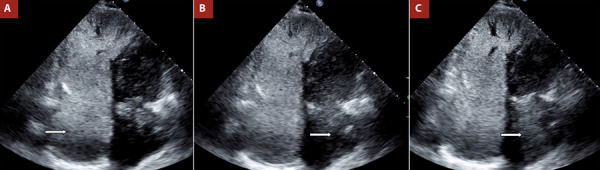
Contrast echocardiography with agitated saline: apical four-chamber view focused on the interatrial septum.
